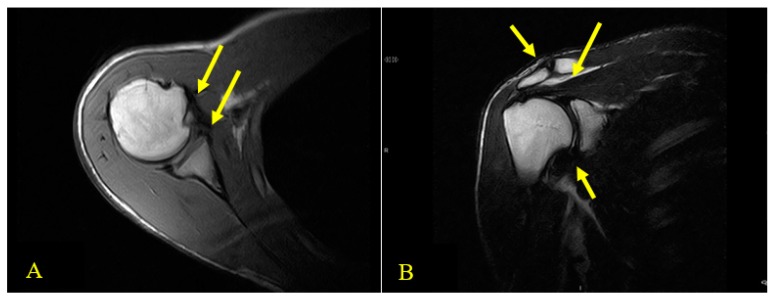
(A) and (B) Axial and coronal views of T1-weighted magnetic resonance imaging (MRI) scans. (A) Axial view of T1-weighted MRI. Two thick yellow arrows indicate inhomogeneity of the antero-inferior portion of the glenoid rim (bottom right), a McLaughlin lesion in the anterior aspect of the humeral head, and an extensive tear of the subscapularis tendon (top right). (B) Coronal view of T1-weighted MRI. Three thick yellow arrows indicate unevenness of the ACJ (top left), lesion of the pre-insertional bundles of the supraspinatus and fluid distension of the subacromial bursa (SAB) with diastasis of the glenoid bone (top right) and, slight thickening of the anterior capsule ligament (bottom right).
